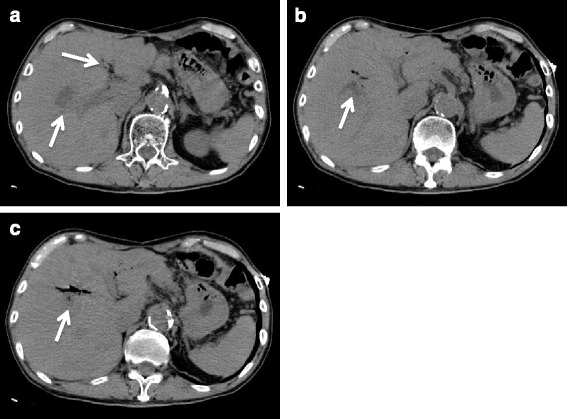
Computed tomography performed on admission. a, b Low-density area with air density detected in segment 8 of the liver (*arrow*). c The *arrow* shows air density in the middle hepatic vein. We determined that the abscess had ruptured into the middle hepatic vein
